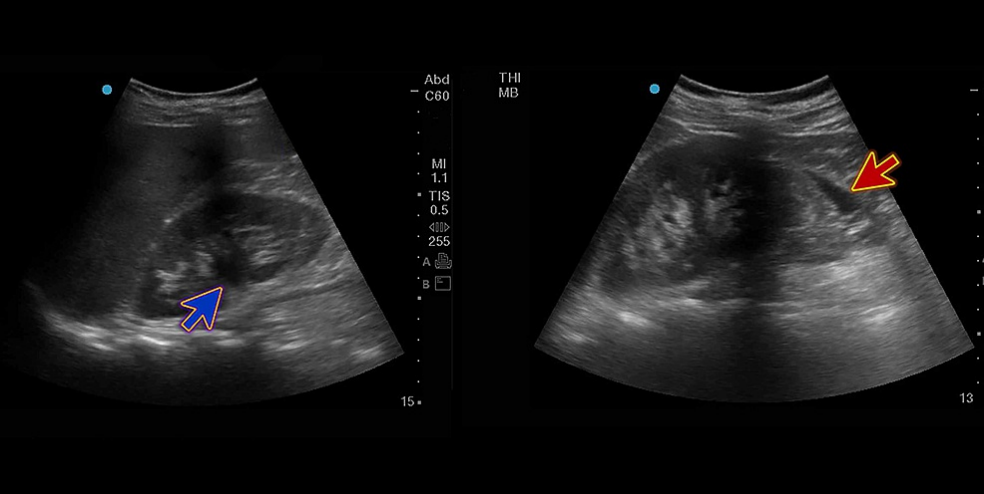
Bedside POCUS showing right-sided mild hydroureteronephrosis (blue arrow) with surrounding perinephric fluid (red arrow). POCUS, point-of-care ultrasound
